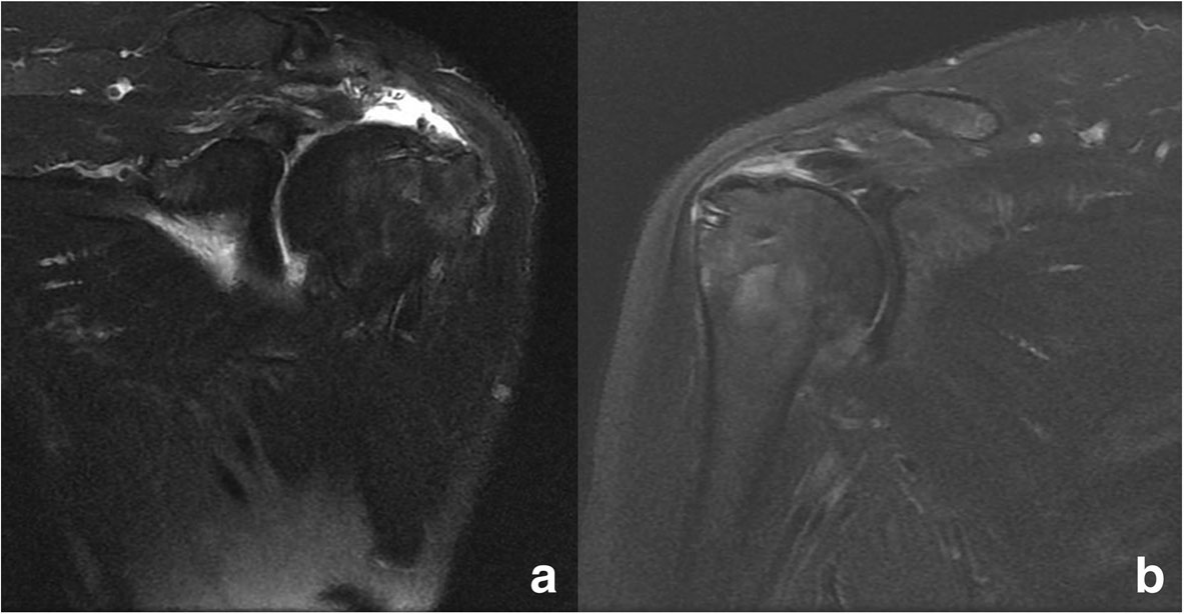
Follow-up T2-weighted sagittal magnetic resonance imaging at 6 months post-operation shows an unhealed tendon of a repaired rotator cuff (type I re-tear). a Conventional suture-bridge technique. b Knotless suture-bridge technique
